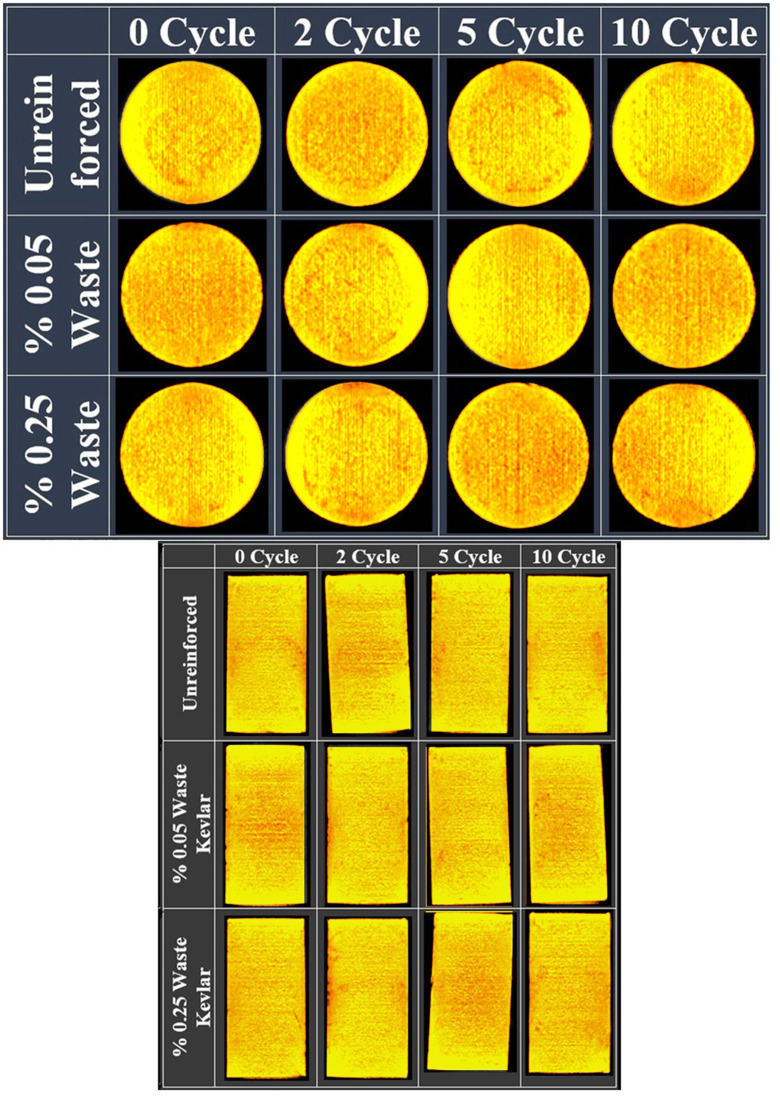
CT scan of CL clay (a- The base of sample’ 21 mm cross section image b- The middle of sample’ longitudinal section image).
